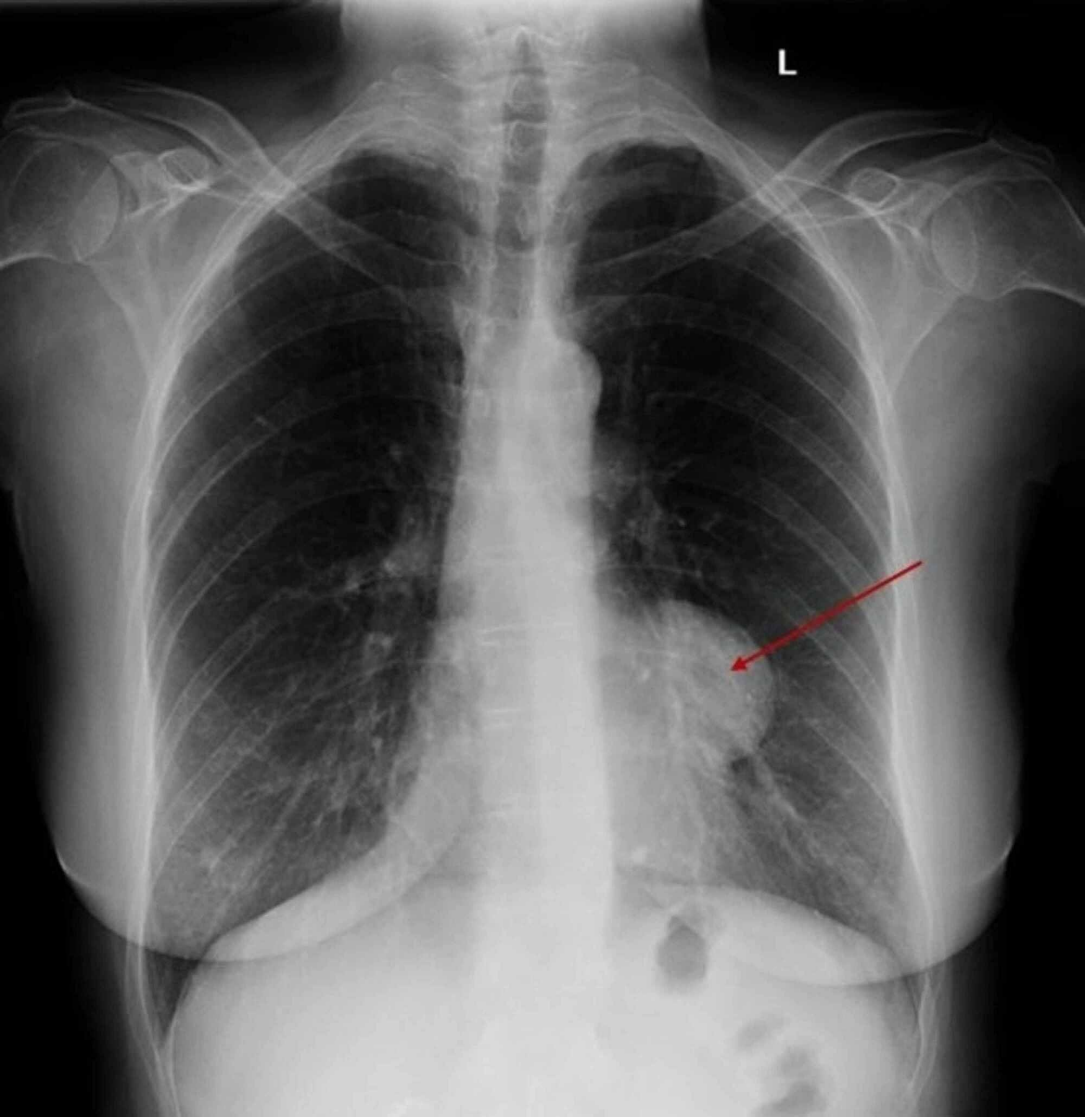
Chest radiograph displaying an anterior mediastinal mass along the left border of the heart.
